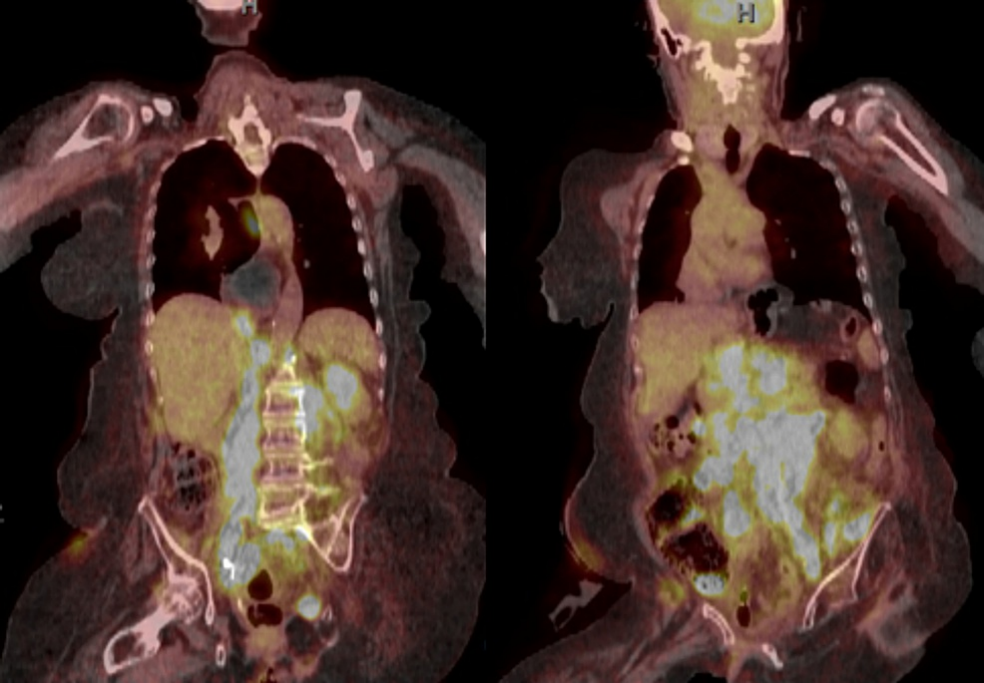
Coronal views, posterior to anterior from left to right, of the FDG-PET status post cystectomy, demonstrating diffuse metastasis at 16 months. FDG-PET, fluorodeoxyglucose-positron emission tomography
